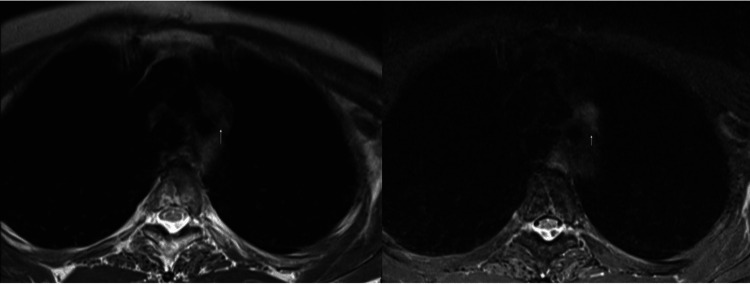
T2-weighted MRI (left) and MRI STIR sequence (right) on December 2021 demonstrating a new mediastinal mass (arrows)
